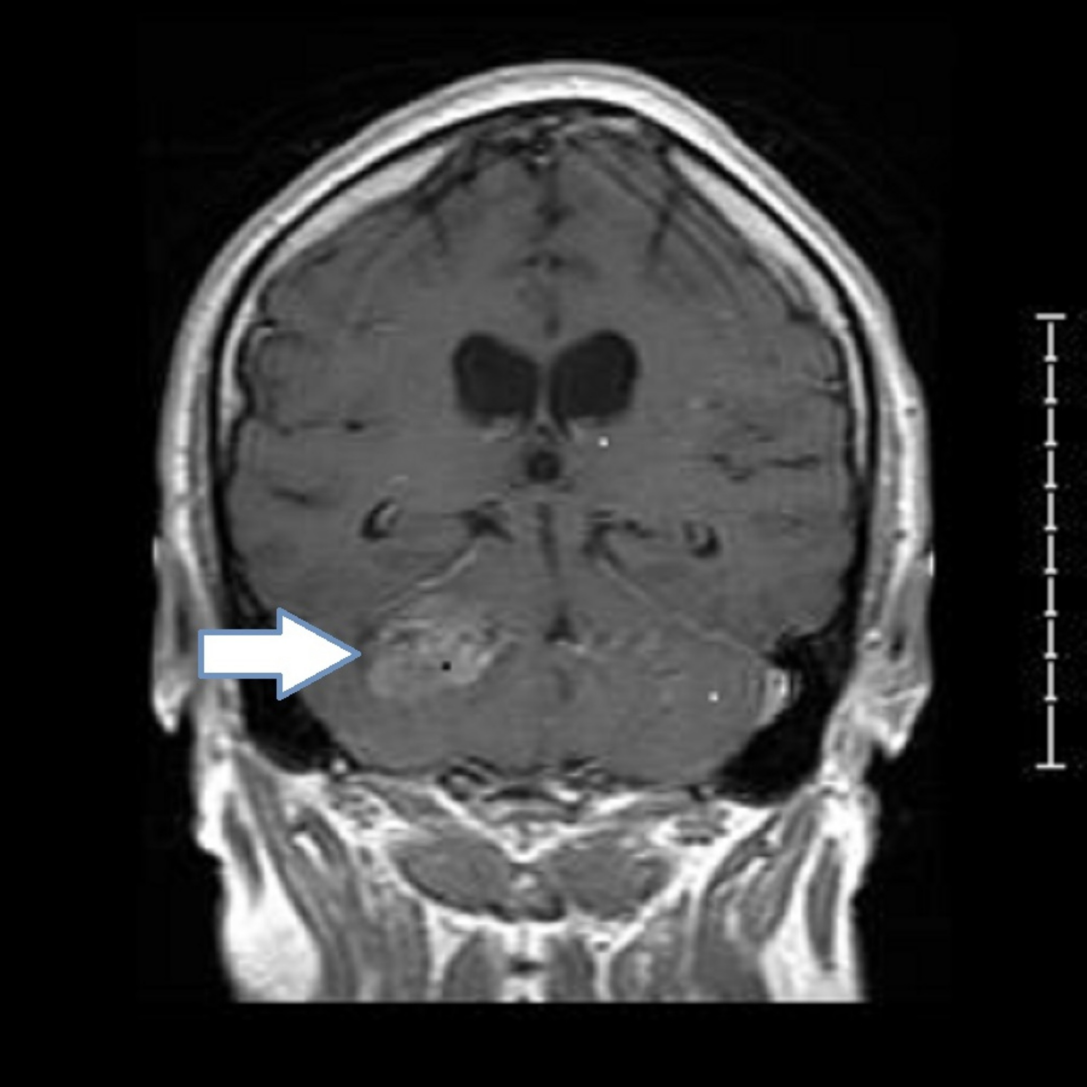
Preoperative MRI of brain with contrast post-COR T1 showing a posterior fossa tumor on the right with asymmetry of the cerebellar soft tissues with increased density
